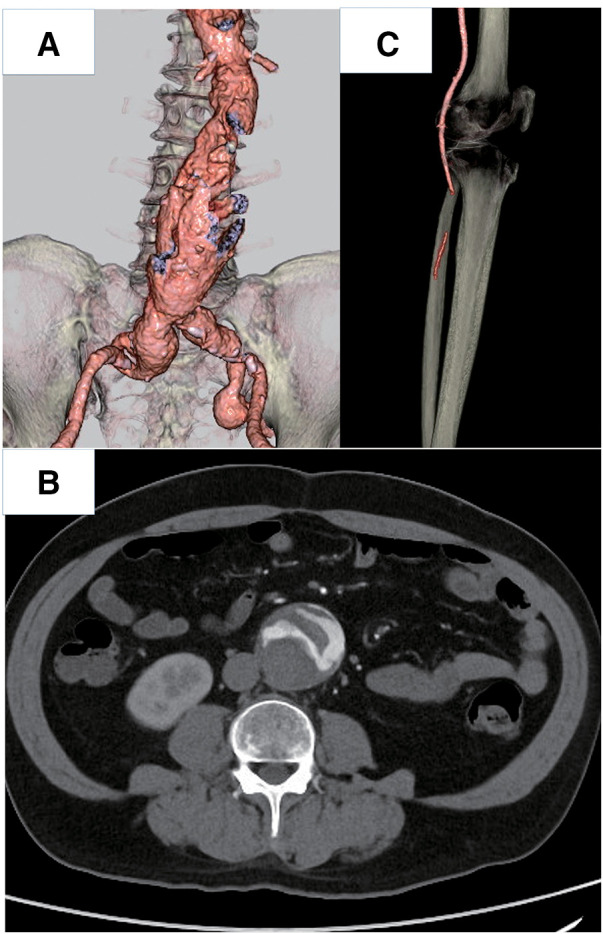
CT scans upon admission. (A) CT angiography of an AAA. (B) An axial image showing heterogeneous mural thrombus in an AAA. (C) CT angiography of the left limb. Arteries below the knee were not detected. CT: computed tomography; AAA: abdominal aortic aneurysm
